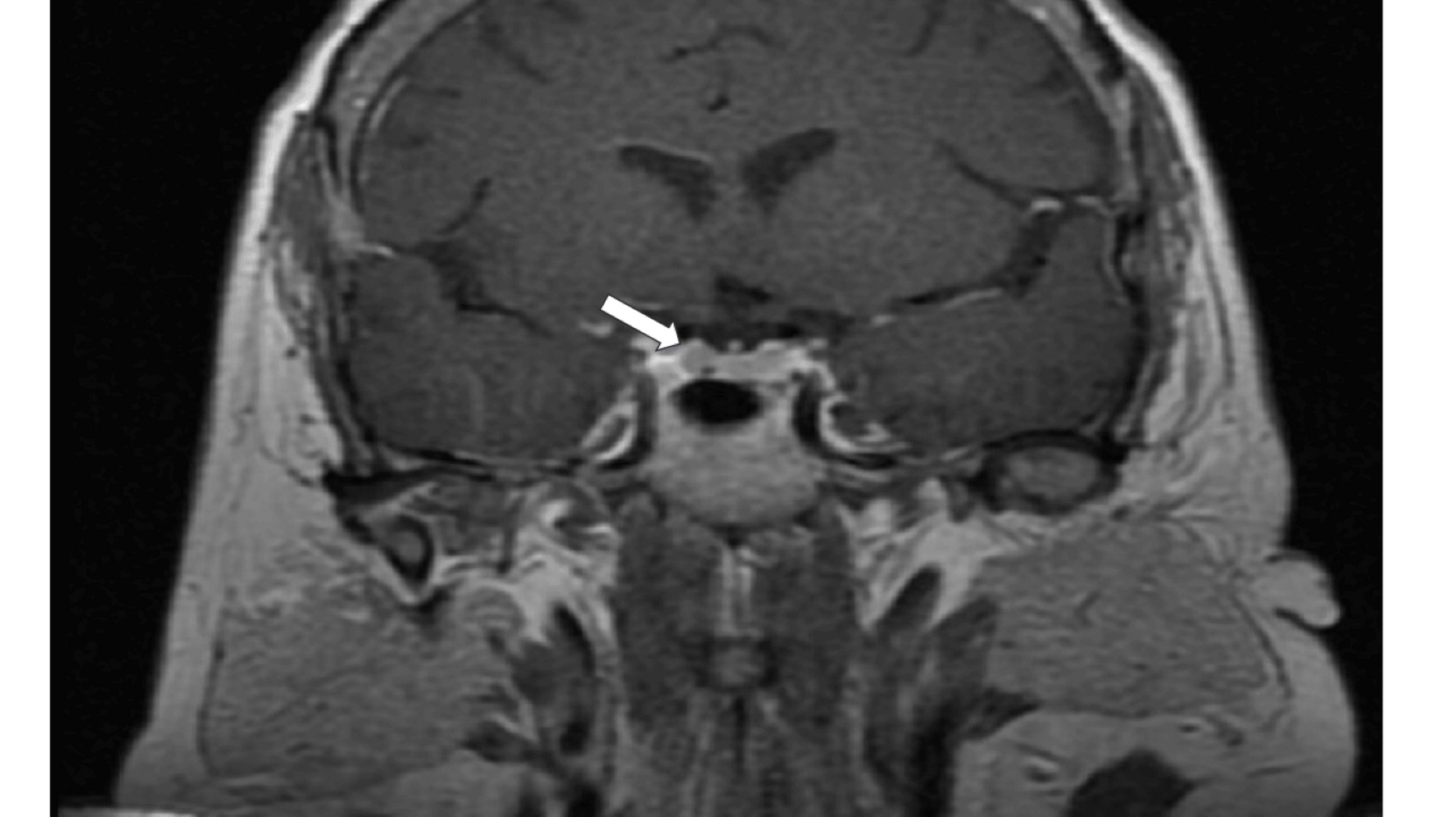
Magnetic resonance imaging (MRI) head with a 4 mm pituitary microadenoma (white arrow).
